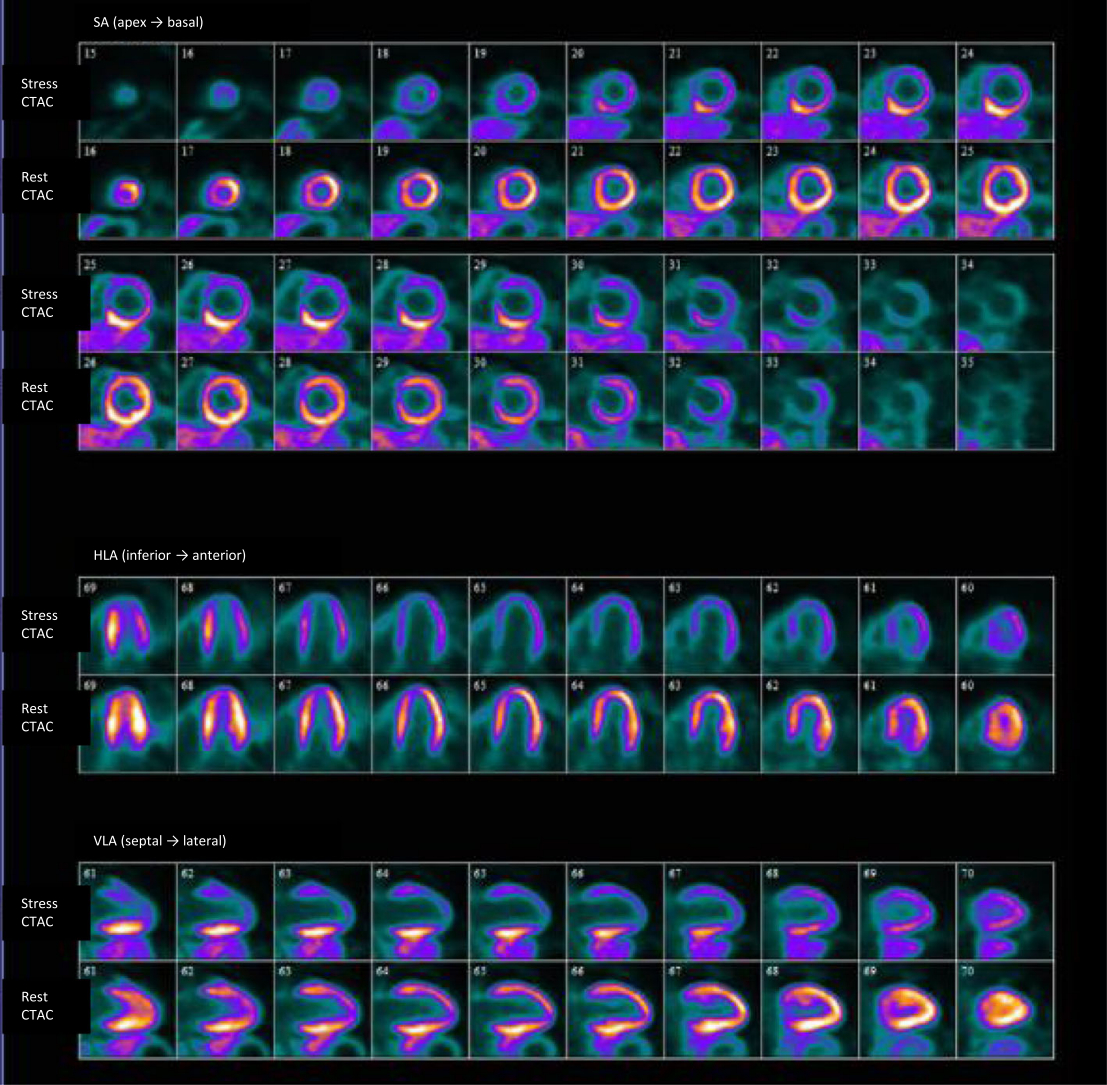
Myocardial perfusion imaging with ^13^N-ammonia positron emission tomography. Stress and rest ^13^N-ammonia perfusion imaging demonstrated significantly decreased uptake in the anterior and lateral lesions and slightly decreased uptake in the inferior lesion. CTAC, computed tomography-based attenuation correction; HLA, horizontal long axis; SA, short axis; VLA, vertical long axis
